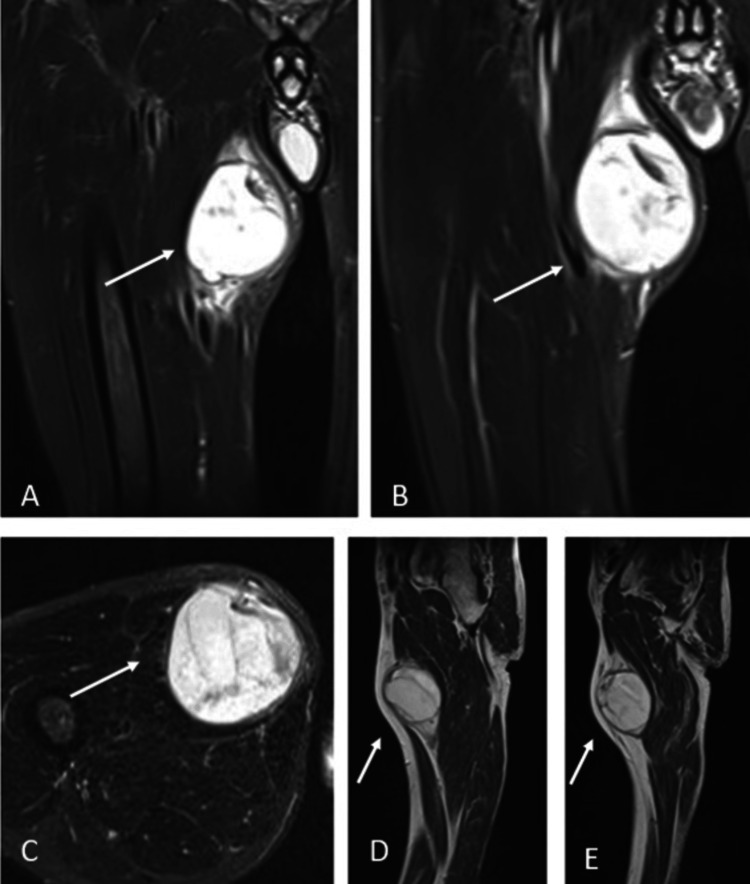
Preoperative magnetic resonance image of the patient can be seen in different sections. The lesion was 60 x 63 x 72 mm in scale. The white arrow shows the lesion in the relevant magnetic resonance images.
